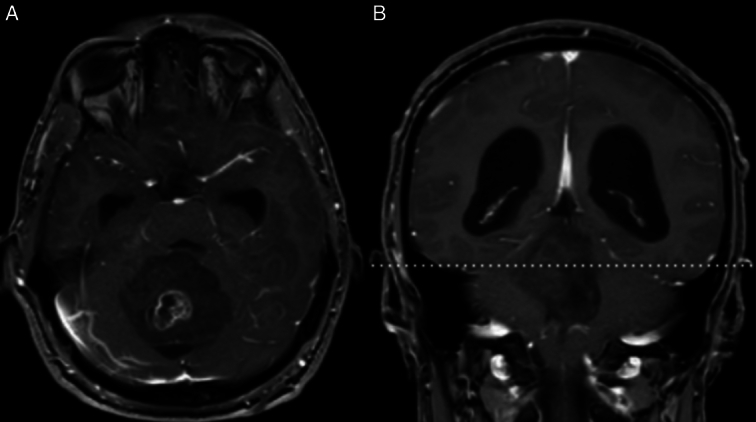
(A) Horizontal T1-weighted MRI scan of the head revealing the presence of a cerebellar mass; (B) coronal T1-weighted MRI scan of the head, highlighting both obstructive hydrocephalus and the cerebellar mass.
